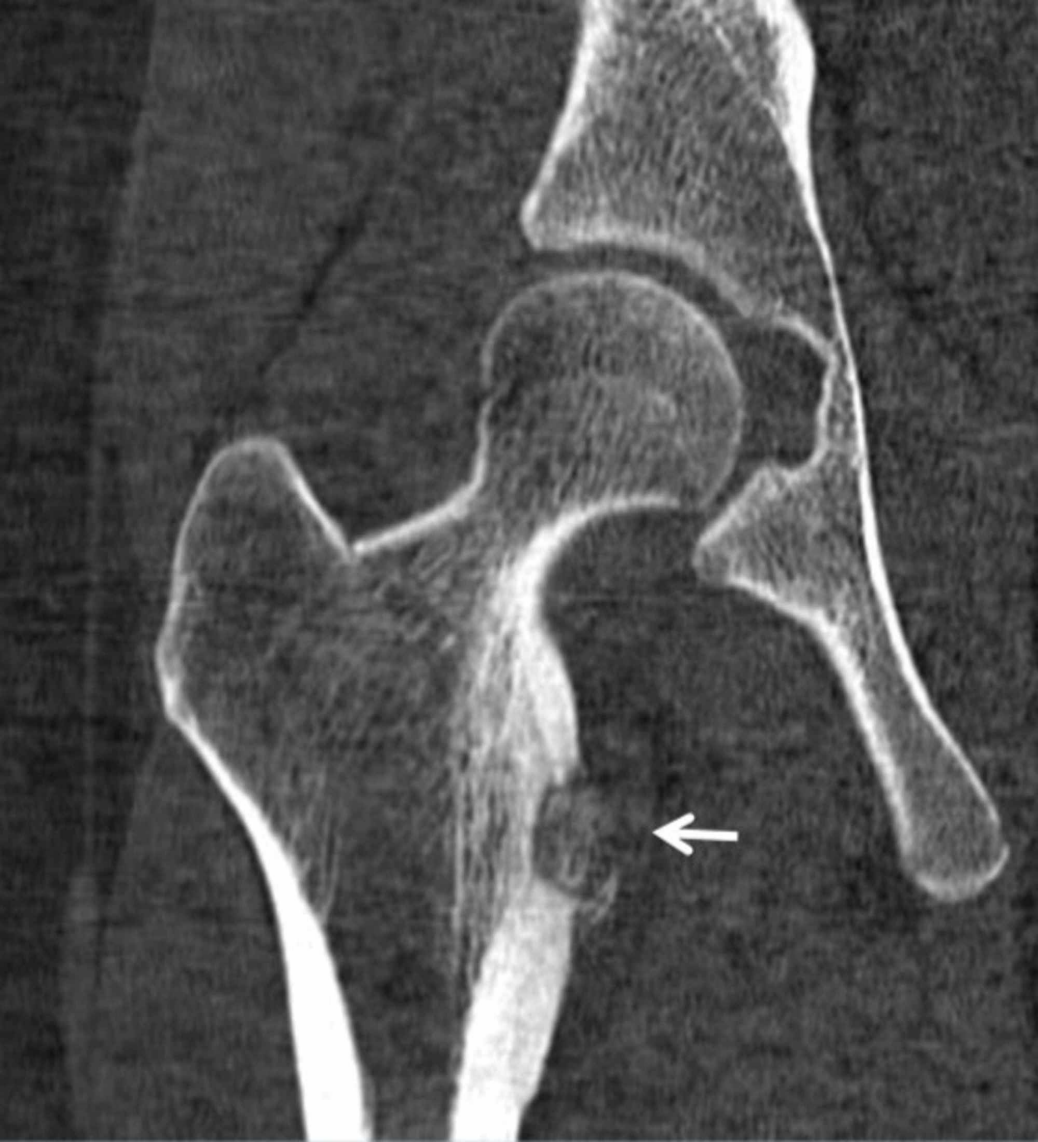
CT image obtained seven months after symptom onset. A 16-year-old female with lytic lesion of the right femoral lesser trochanter cortex. Coronal CT image obtained seven months after symptom onset shows a cortically based lytic lesion (arrow) with adjacent periosteal reaction and adjacent reactive sclerosis. No presence of an internal sclerotic nidus. The lesion has internal osteoid matrix with extra osseous matrix extension in the medial soft tissues at the iliopsoas tendon insertion.
